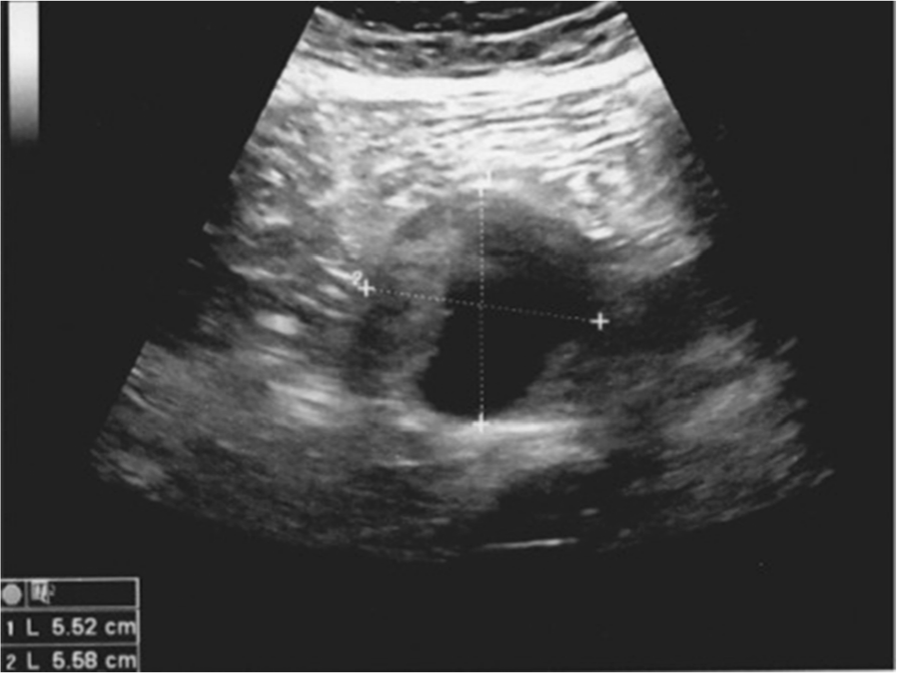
Ultrasound image of the tumour
